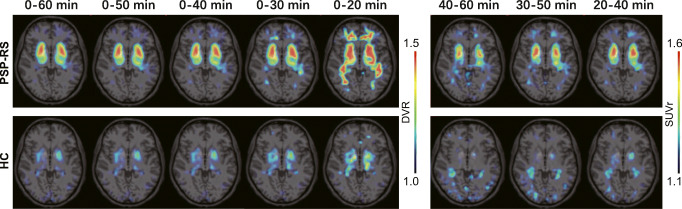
Representative [^18^F]PI-2620 images for different dynamic and static imaging windows. Axial slices upon an MRI standard template of a patient with PSP Richardson syndrome (PSP-RS; female, 69 years, PSP rating scale: 34) and a healthy control (HC; female, 70 years) show distribution volume ratios (DVR) and standardized uptake value ratios (SUVr)
